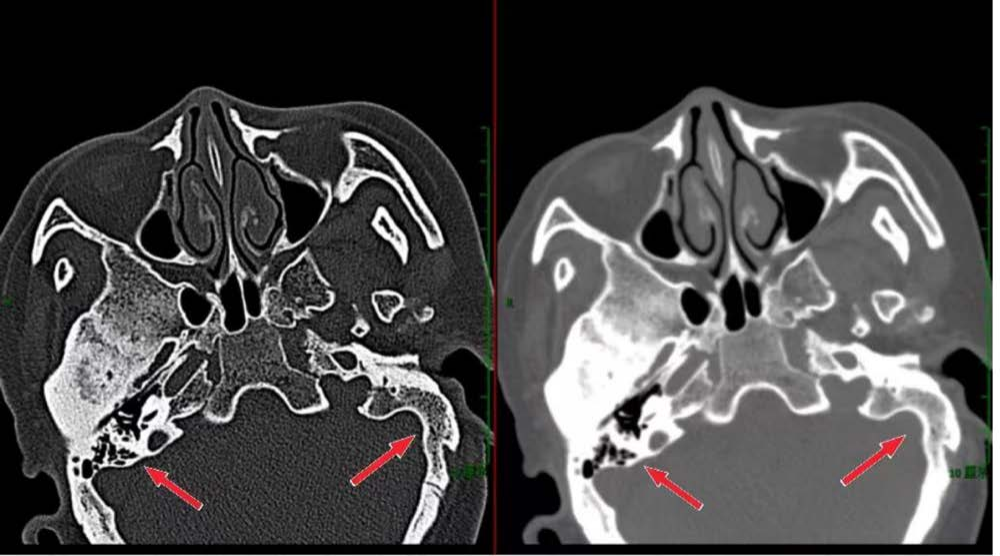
Mandibular bone/section (spiral) (CT imaging + 3D reconstruction): Temporomandibular joint osteoarthritis on both sides, with significant symptoms on the right side. CT = computed tomography.
